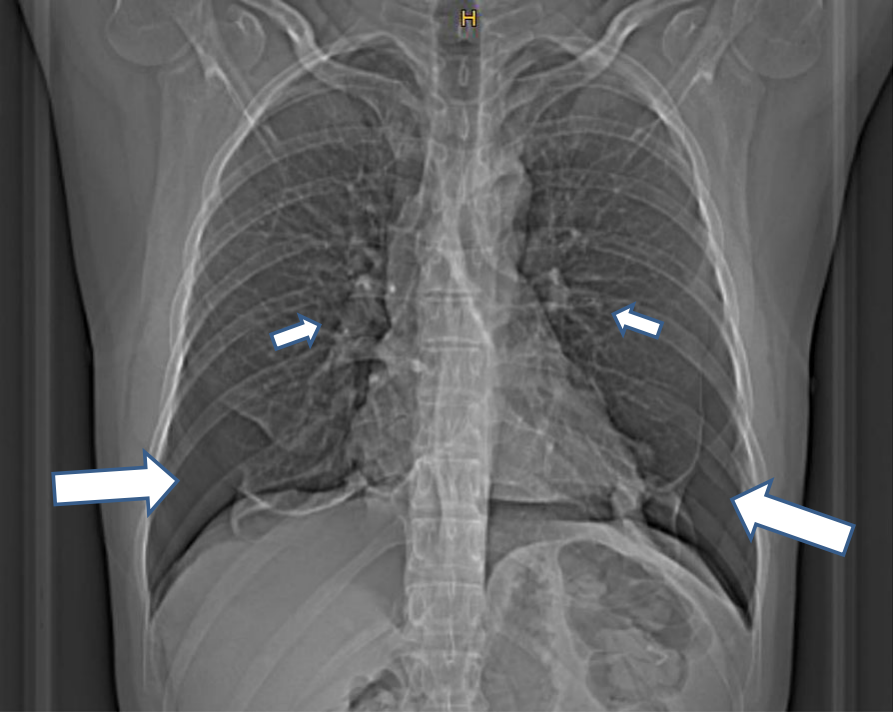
Chest computed tomography scan from the index patient. Bilateral pneumothorax (large arrows) and lung bullae (small arrows) are indicated. The pneumothorax is mostly localized in the basal parts of the chest cavity due to previous pleurodesis treatment
